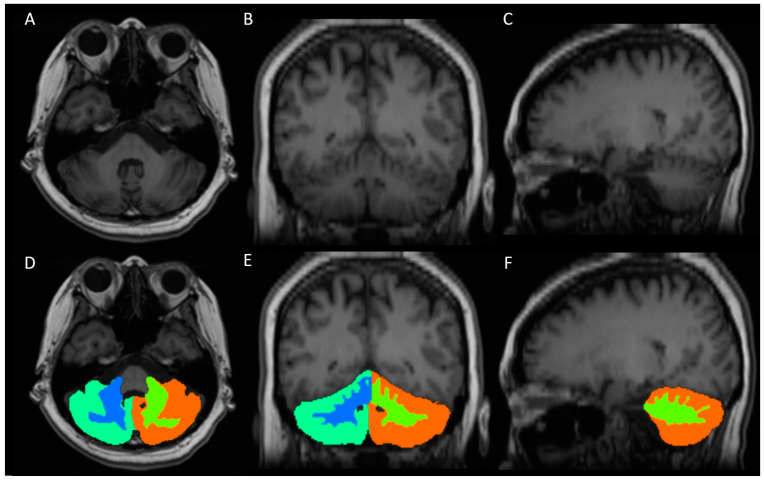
A representative case of cerebellar segmentation. (A–C) Axial, coronal, and sagittal view of T1-weighted high-resolution image, respectively. (D–F) Axial, coronal, and sagittal view of right cerebellar gray matter mask (cyan), right cerebellar white matter mask (blue), left cerebellar gray matter mask (orange), and left cerebellar white matter mask (green), respectively.
